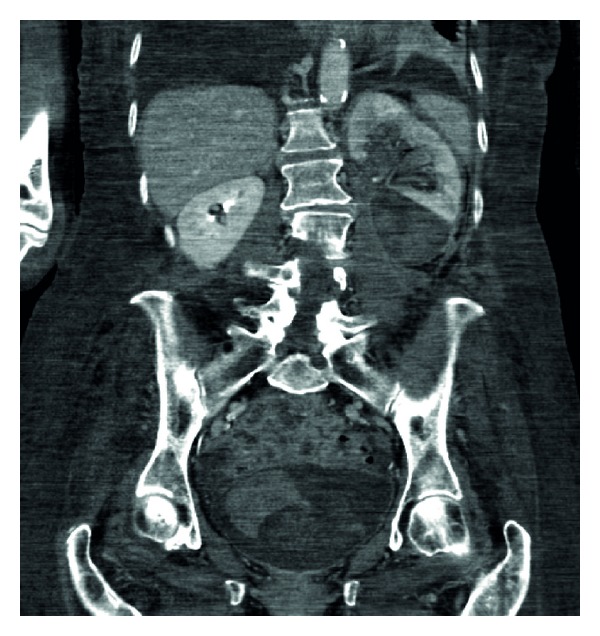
Split bolus contrast CT KUB: coronal section.
